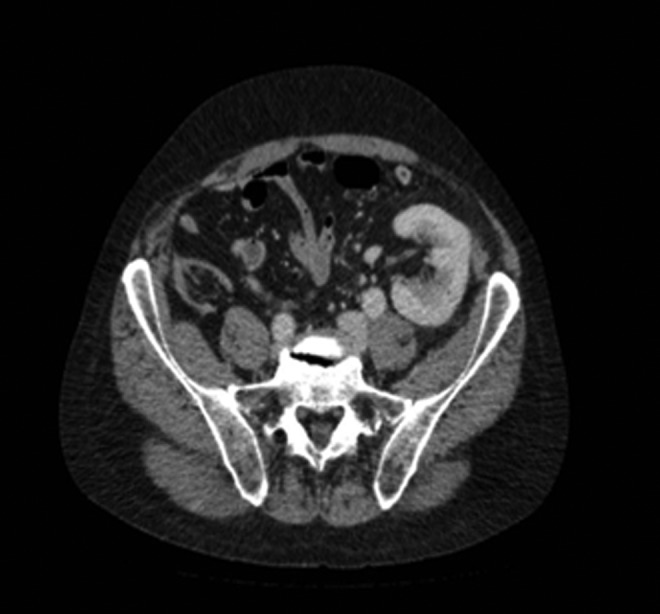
Case 2—CT urogram; axial image with renal transplant located lateral in pelvis.
